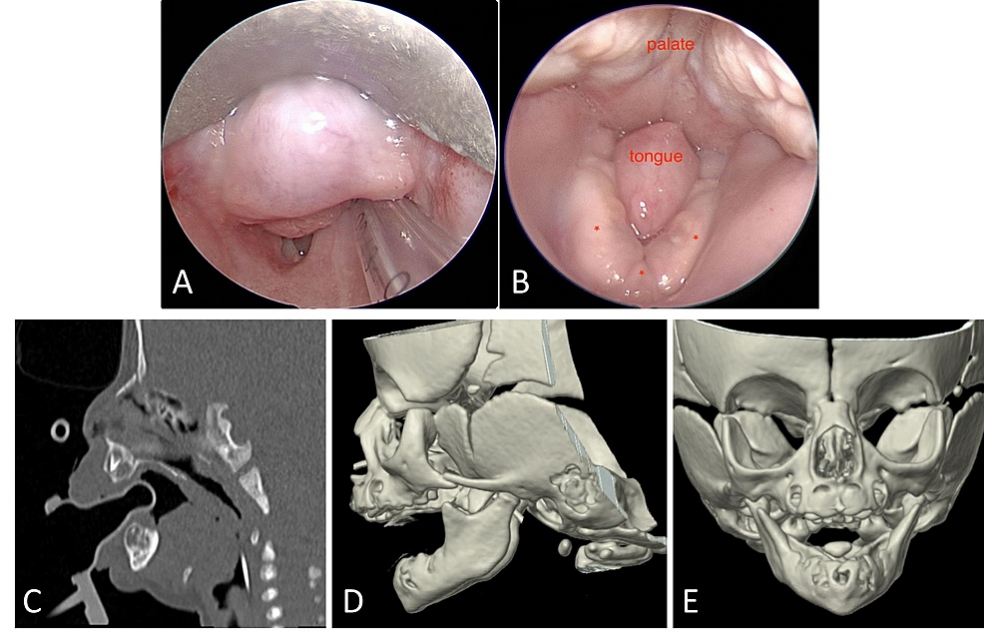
Case 1 (A) Short rudimentary tongue filling the narrow space between the right and left mandibular bodies. (B) Direct laryngoscopy revealing the glossoptosis and supraglottic obstruction. (C) Computed tomographic scan of the two-week-old infant in the sagittal plane with soft tissue window. Note the absent anterior tongue and its posterior part causing glossoptosis obstructing the upper airway. (D) 3D view of the hypoplastic mandible and condyles. (E) 3D view of the transverse deficiency.
